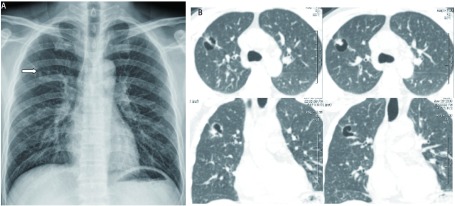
图A、B分别为病例2的胸片及胸部CT表现。A：胸片见右肺上叶一空腔性病变；B：胸部CT见右上肺含壁结节的空腔，空腔周围可见毛刺及胸膜凹陷征。 Pictures A and B are the shows of radiograph and CT scan of case two. A: Chest X-ray shows a cavity in the right upper lobe; B: CT shows a cavity with mural nodule in the right upper lobe. Radioactive burr and pleural indentation sign can be found around the cavity.
